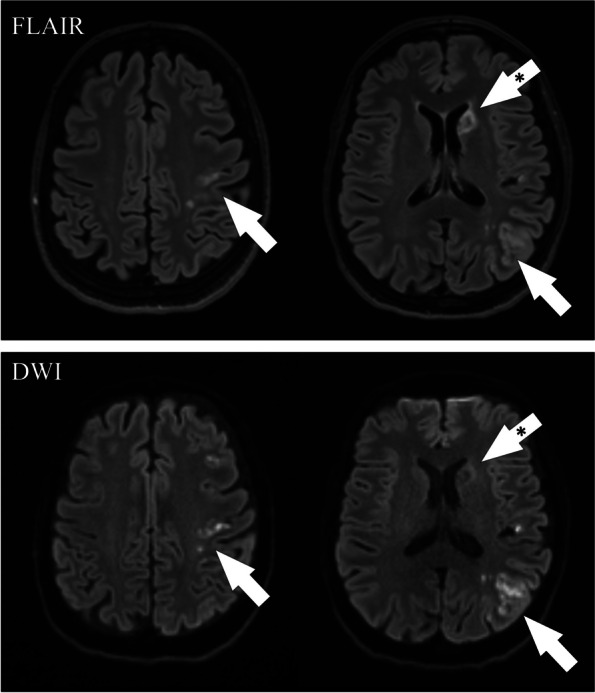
MRI on Fluid-attenuated inversion recovery (FLAIR) and Diffusion-weighted sequences showing multiple asynchronous lesions in the territory of the left middle cerebral artery (white arrows) and a subacute/chronic lesion of the head of the caudate nucleus, in the territory of the recurrent artery of Heubner (asterisk-marked arrow). All the ischemic lesions are in the left internal carotid artery territory
